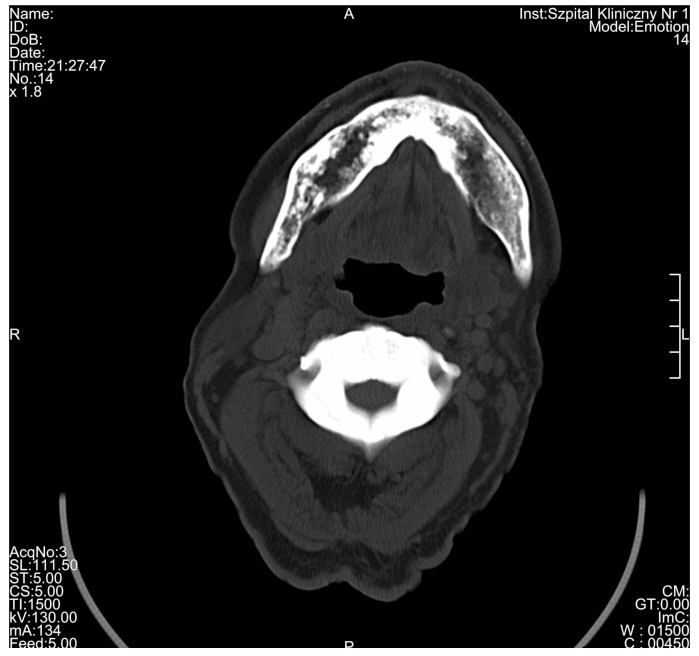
Significant osteosclerosis of the mandible and axis in 80-year-old patient suffering from osteopetrosis visible on cross-sectional computed tomography scan.
